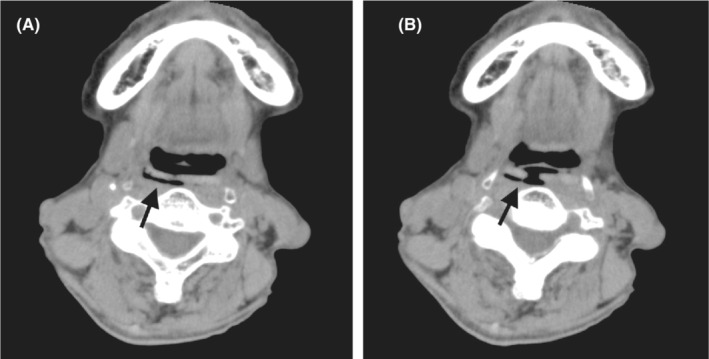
(A) Coronal view of computed tomography imaging showing the presence of air along the posterior wall of the pharynx (arrow). (B) Coronal view of computed tomography imaging showing the presence of air along the posterior wall of the larynx.
